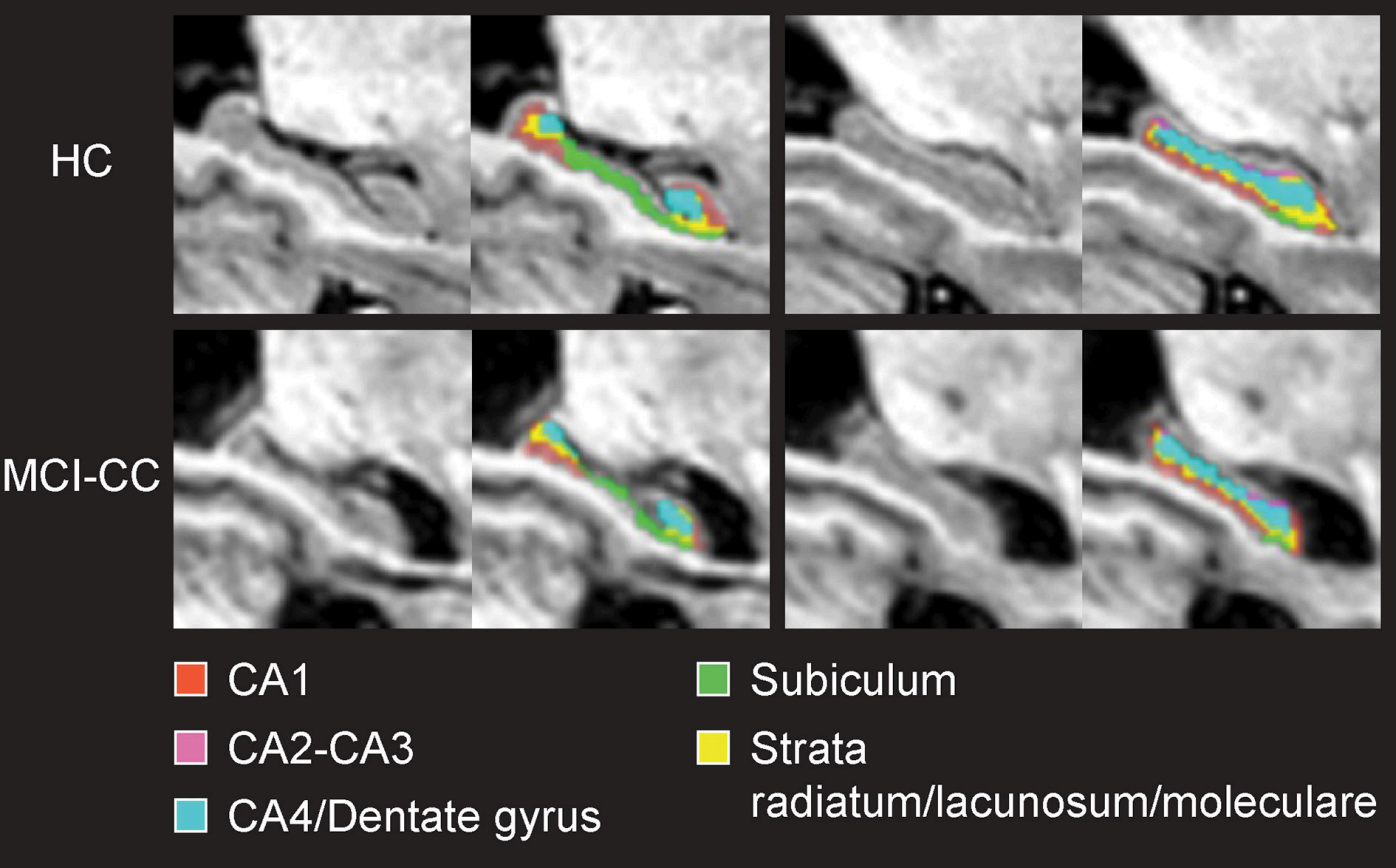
Coronal views of the hippocampus and hippocampal subfields in magnetic resonance images from a healthy control subject (HC) and a mild cognitive impairment converter subject at time of conversion (MCI-CC) of the present study.
